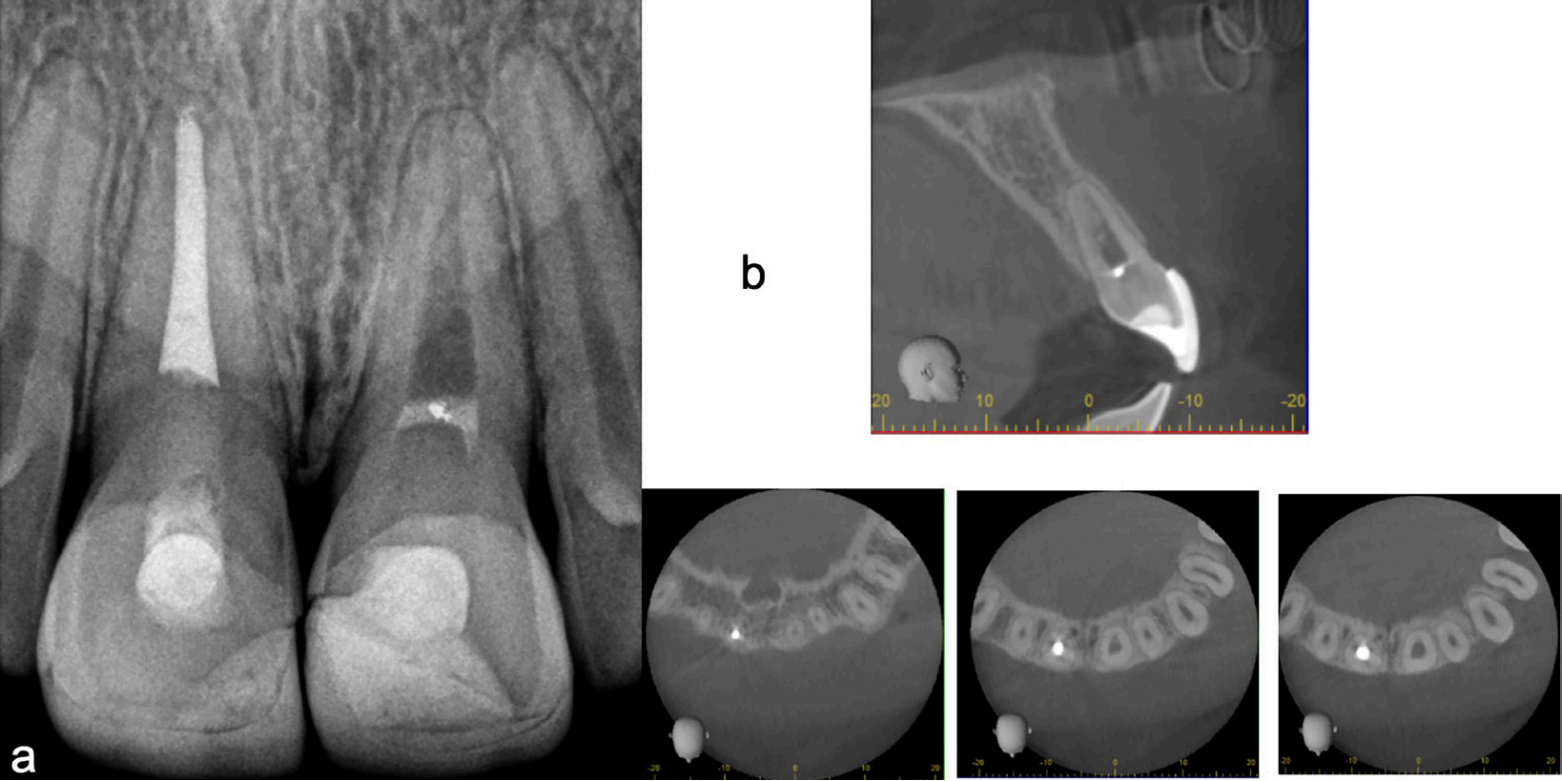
Late radiographic follow-up demonstrating continued root maturation and apical healing of tooth 21. (a) Eight-year follow-up periapical radiograph showing marked increase in root length and thickening of dentinal walls, consistent with progressive root maturation. (b) Ten-year follow-up CBCT confirming apical closure, absence of periapical lesions, and increased thickness of the root canal walls, supporting long-term apical healing and root development.
